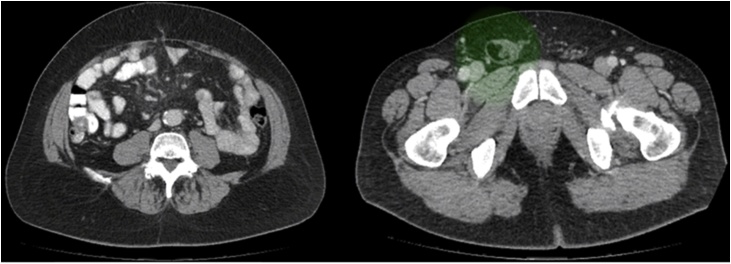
CT Abdomen: right inguinal hernia and umbilical hernia.
